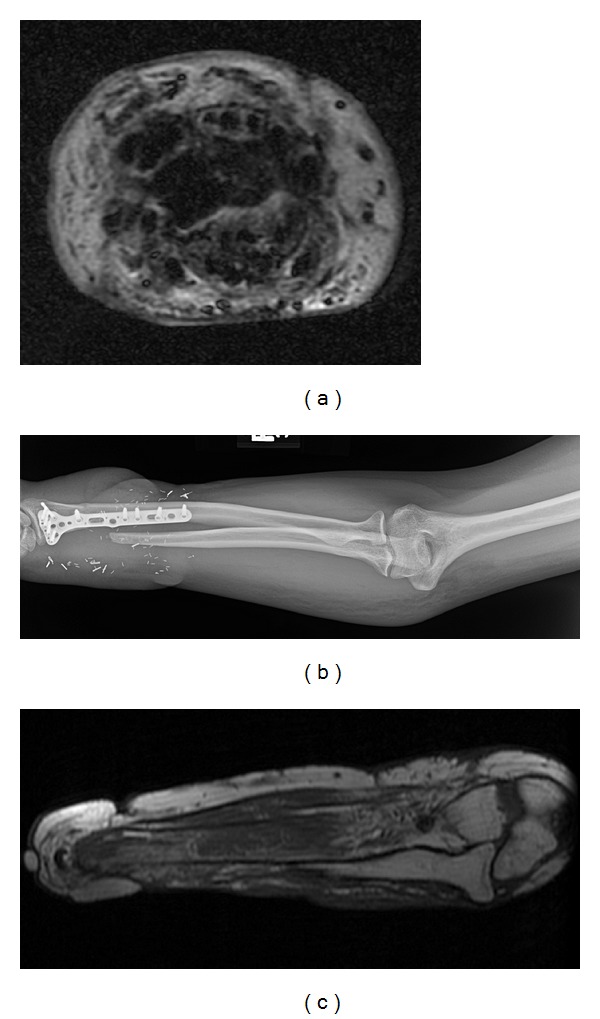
(a) Axial IR MRI without contrast of the extremity shows circumferential edema and skin thickening consistent with cellulites. This patient underwent antibiotic therapy prior to transplantation. (b) Corresponding radiograph showing extensive soft tissue edema. (c) MRI of a different patient demonstrated diffuse muscle atrophy. This patient was disqualified from consideration.
